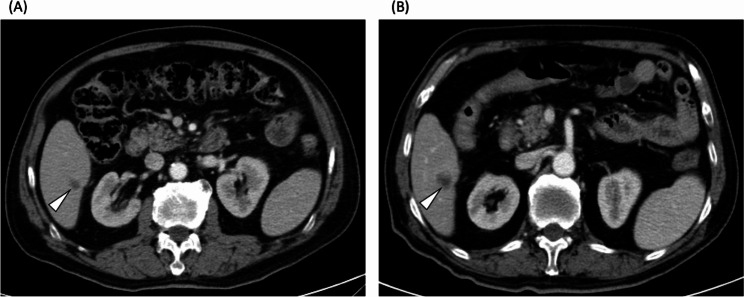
A 86-year-old with well-differentiated adenocarcinoma of sigmoid colon with liver metastasis. Before initiation of bevacizumab-based chemotherapy (A), CT scan showed a 1.1-cm metastatic lesion (arrowhead) in the segment 6 of liver. After three months of treatment with bevacizumab-based chemotherapy, CT scan (B) demonstrated increased size (1.6 cm) of this metastatic lesion (arrowhead) with indistinct liver-tumor surface. RD-MR defined this patient as a non-responder. In ResNet-MR, this patient was classified as a responder. The patient survived for 31 months after initiation of bevacizumab-based chemotherapy
